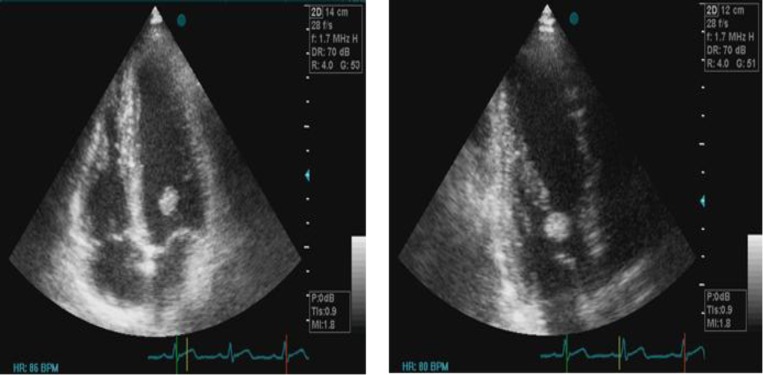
A, B Echocardiography (Four chamber and three chamber view) showed a bulbar mass of 10 15 mm attached at mitral valve anterior leaf let (a white arrow).
